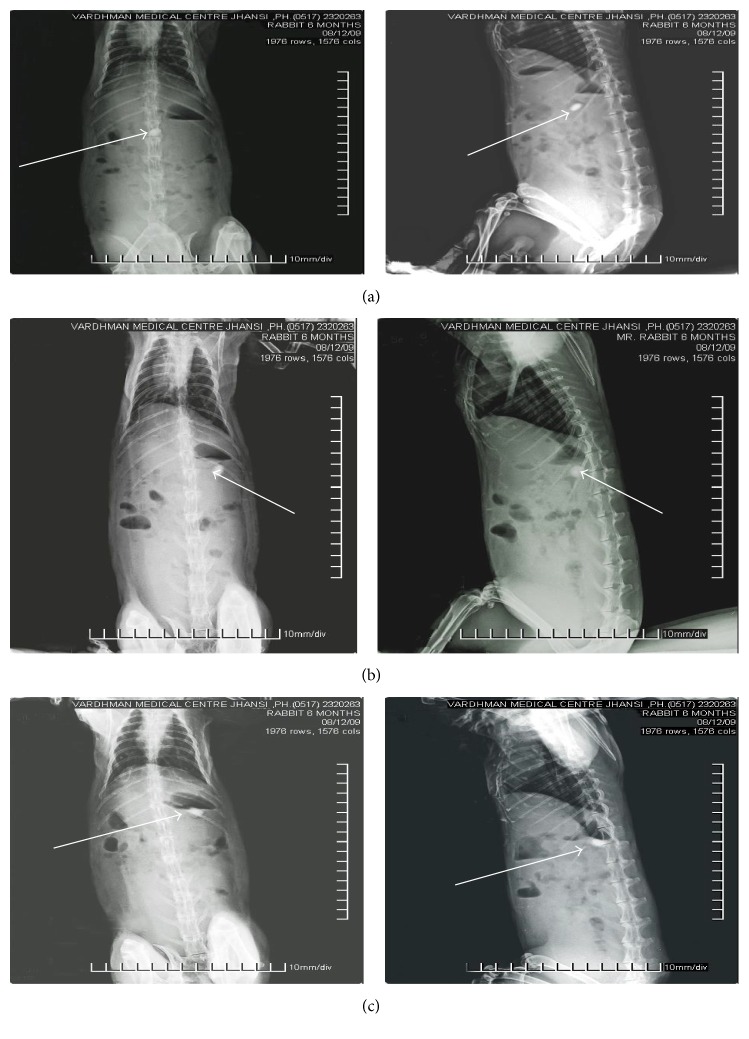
Radiographic images showing the presence of BaSO_4_ loaded floating tablet in the stomach at different time periods (the tablet is indicated with an arrow). Images were taken after (a) 1 hr, (b) 3 hr, and (c) 6 hr, after tablet administration.
